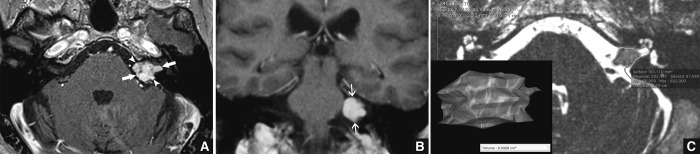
MRI measurements. A and B) The largest anteroposterior diameter (arrowhead) and mediolateral diameter (large arrows) were measured on axial section (A). The largest superoinferior diameter (thin arrows) was measured on coronal section. Anteroposterior and superoinferior diameters concerned cerebellopontine angle (CPA) extent only. Mediolateral diameter included both CPA and internal auditory meatus (IAM) extent. C) Volumetric measurements were performed as shown by contouring the tumor in every axial section on HRT2-weighted images. Volume was then calculated automatically using OsiriX software.
